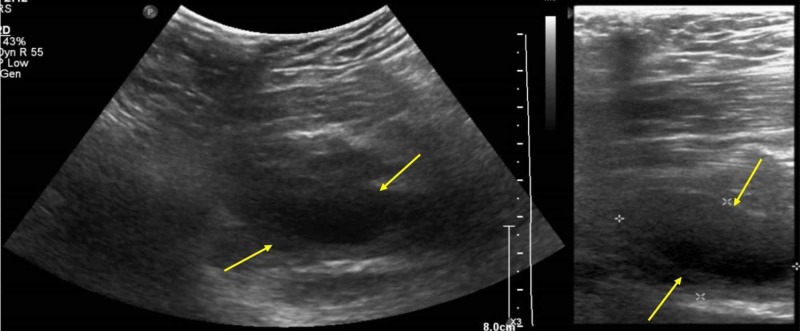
Soft tissue ultrasound scan: collection of fluid within the left adductor muscle and early cellulitis.
